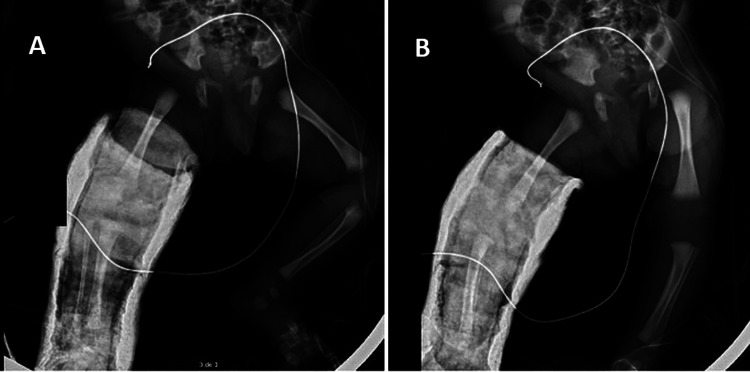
Radiographs of the right lower limb after a plaster cast at 5–10° A: anteroposterior; B: lateral views.
